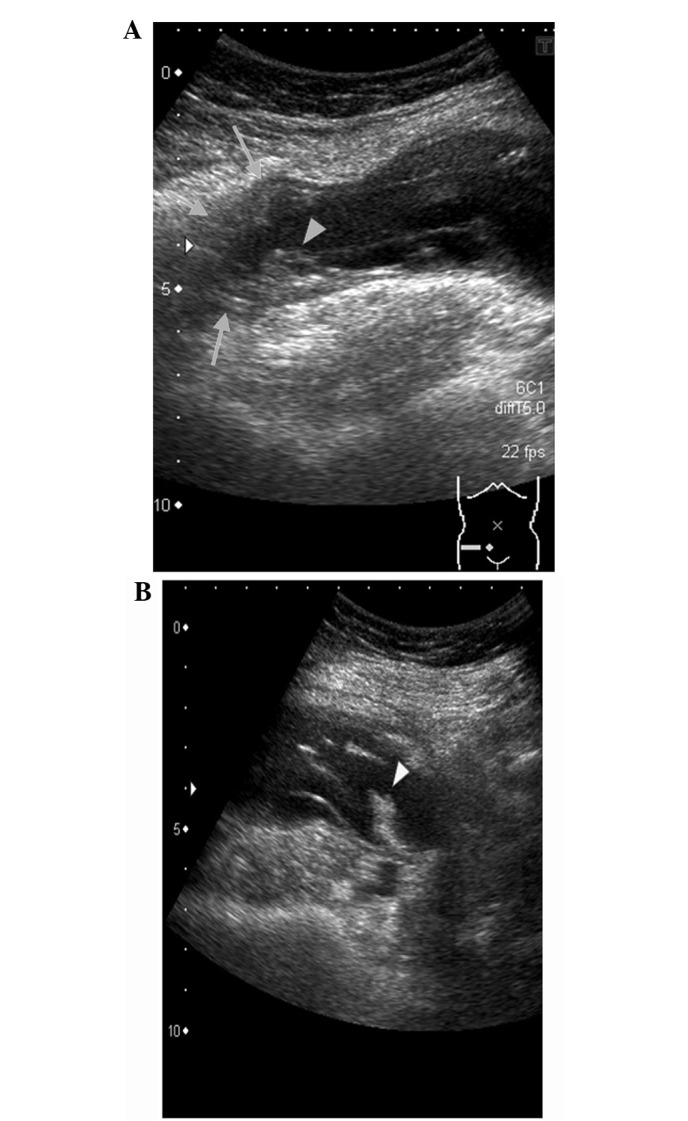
Abdominal ultrasonograms obtained in August 2012. An anechoic mass with a partly layered echo pattern was visible from the appendicular ostium (A) and from the appendicular tip (B). The mass wall on the appendicular ostium was thickened (A, arrow), with a 13-mm projection protruding toward the cavity from part of the wall. Another 9-mm projection was visible on the appendicular tip (B, arrowhead).
